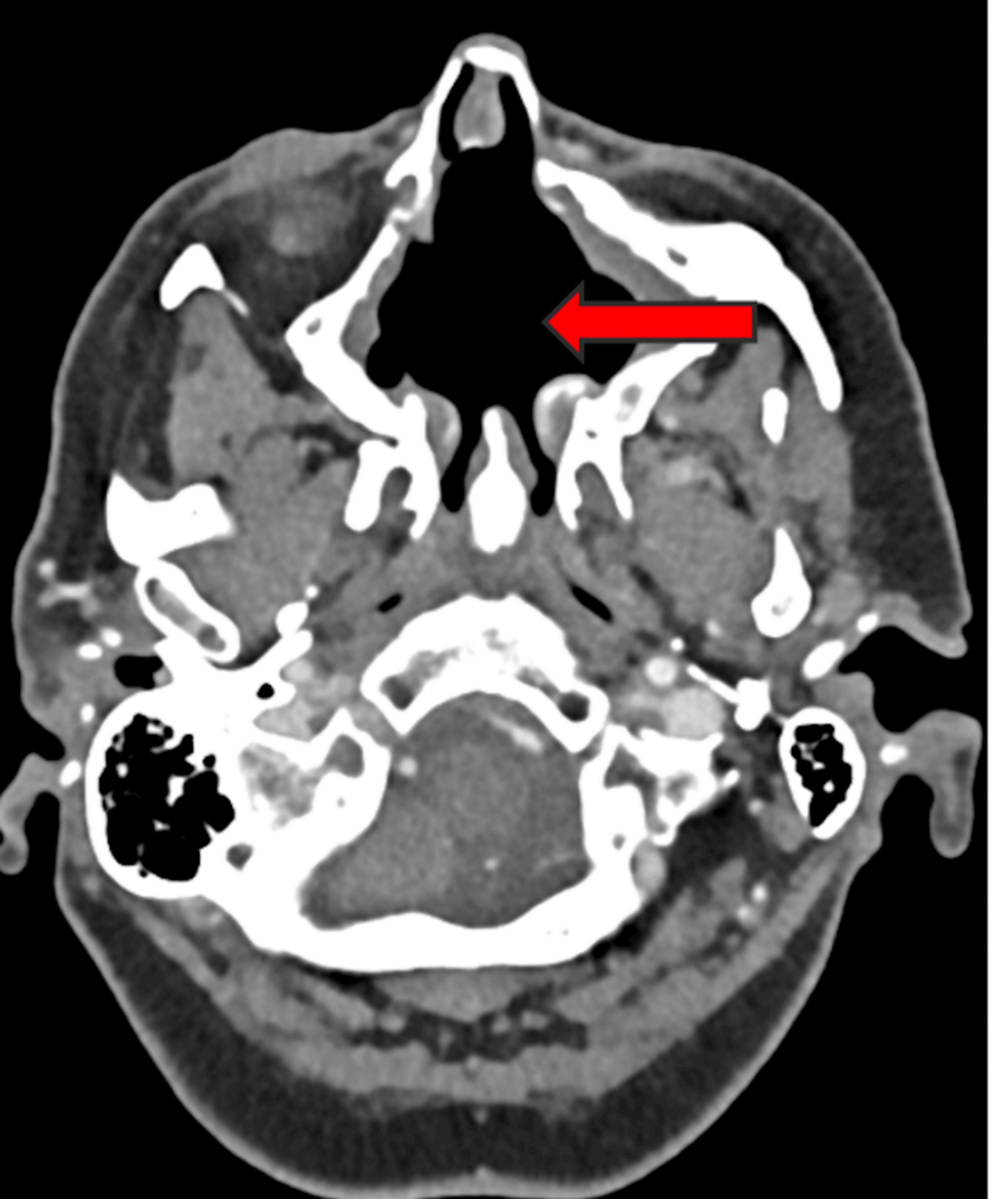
Postoperative contrast-enhanced CT scan of the paranasal sinuses The image demonstrates postoperative changes after subtotal maxillectomy (red arrow) with restoration of the sinonasal anatomy and preservation of the orbital floor. No residual or recurrent enhancing lesion is seen CT: computed tomography
